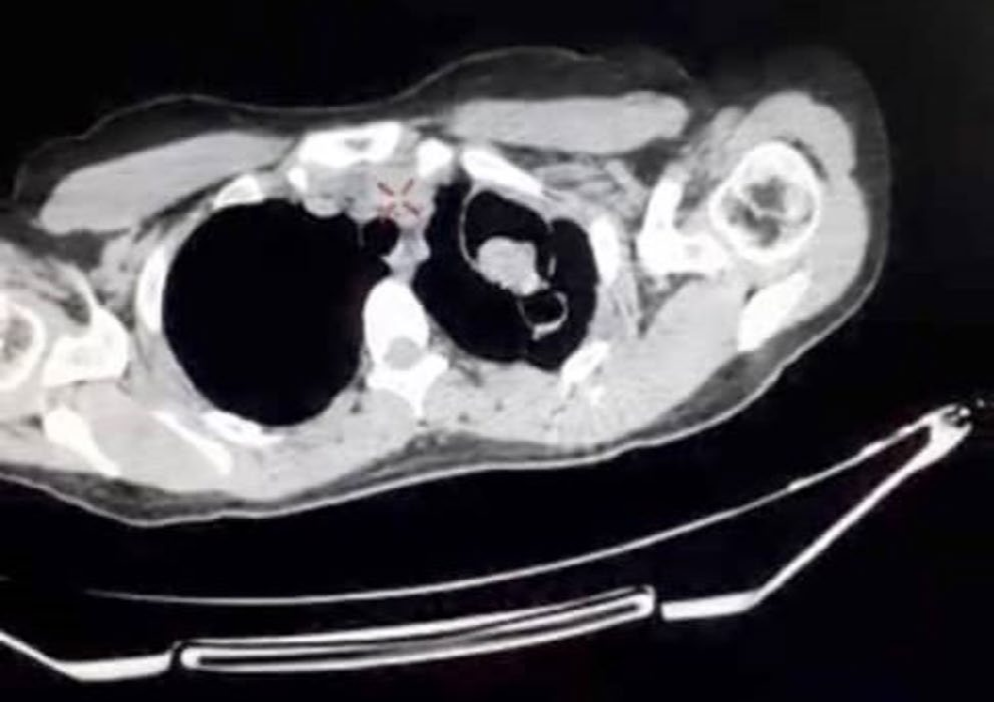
Axial chest CT scan (mediastinal window) revealing a well‐defined cavitary lesion in the left upper lobe with an internal round structure resembling a fungal ball.
